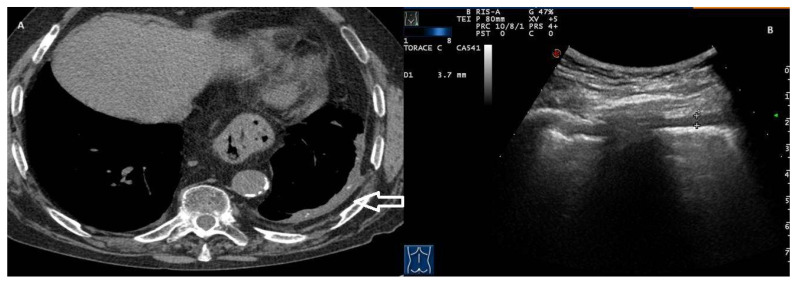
(A) Axial CT image with soft tissue window documents left, pleural thickening with calcifications and expansion of extrapleural fat (arrow); these features are suggestive of benign pleural fibrosis. Note the presence of a hiatal hernia. (B) Transthoracic ultrasound scan with a convex probe (7 MHz) corresponding to pleural fibrosis in the CT image, which shows an irregular hypoechogenic pleural thickening (3.7–5 mm). Videoclip 2 also shows the absence of a gliding sign with the presence of a “lung point”.
